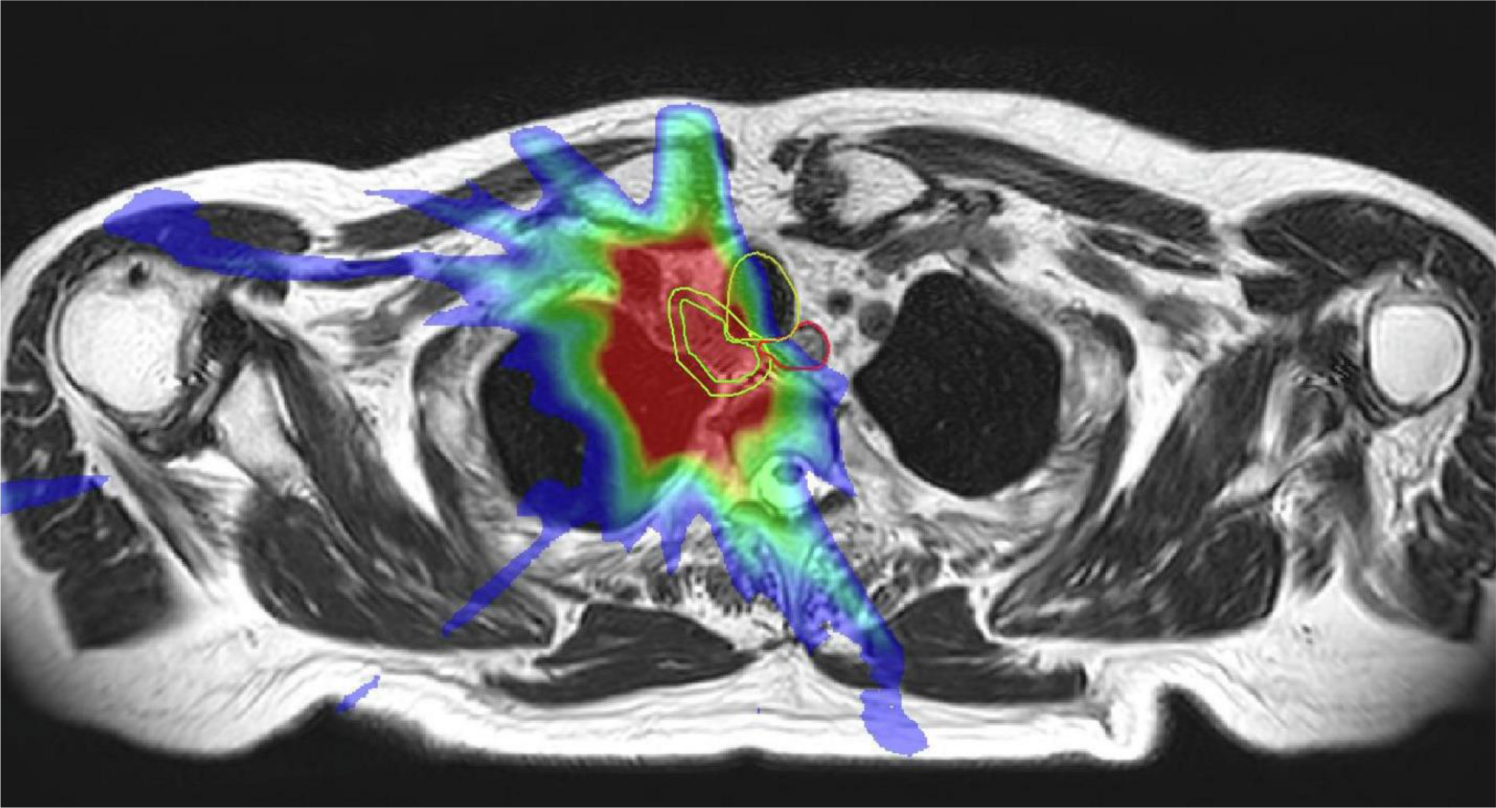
Online MRI and physical dose distribution of a patient with a mediastinal lymph node metastasis of a SCLC in whom a 58% decrease in ITV occurred. The dose distribution of the original plan is projected on the reduced target size at the 12th fraction The ITV (inner green line) and OARs (oesophagus, trachea, spinal cord) within a 3 cm expansion (yellow) around the ITV were modified each fraction by a radiation oncologist. Reprinted with permission from ref. 37. Copyright 2024, Elsevier.
